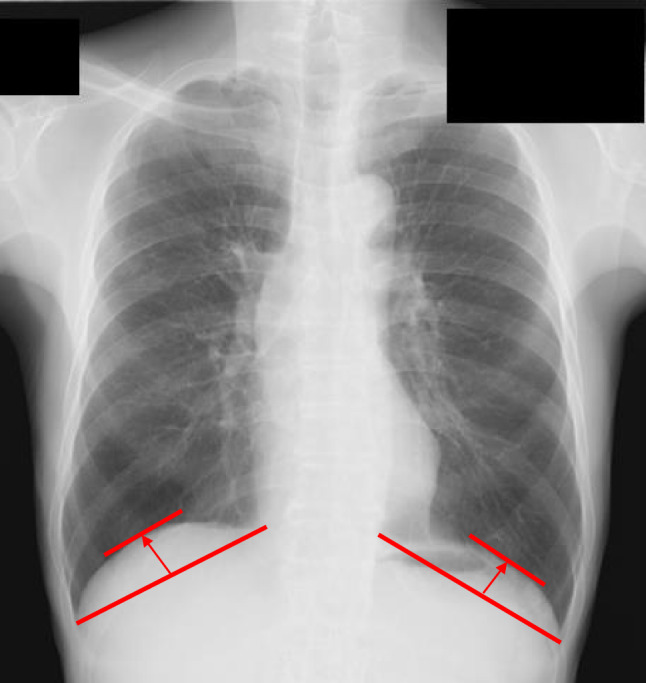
Measurement of diaphragmatic dome height. The height of the right and left diaphragmatic domes was measured using plain chest radiographs acquired within 1 month preoperatively. The measurement was defined as the longest vertical distance from the diaphragmatic silhouette to a line connecting the costophrenic and cardiophrenic angles
